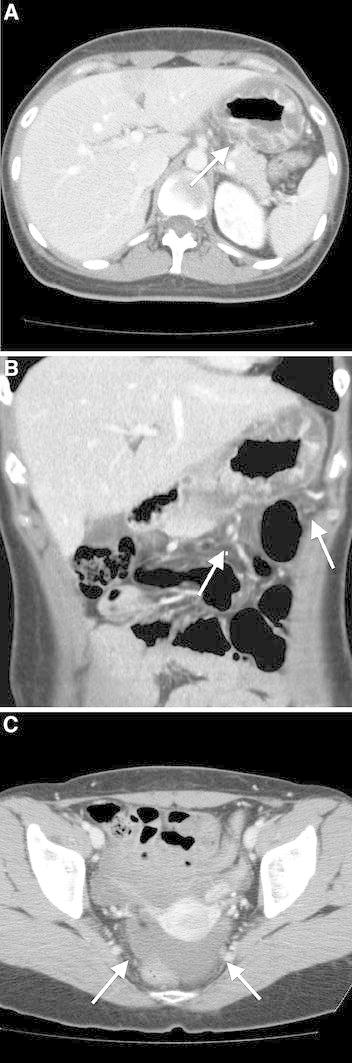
Gastric anisakiasis in a 32-year-old woman who presented with severe epigastric pain and a history of eating raw fish 1 day before symptom onset. A The CT image shows the severe mucosal edema of the whole gastric wall (*arrow*). B The coronal reconstructed image shows the edematous change of the stomach. Fat infiltration was also seen around the stomach (*arrow*). C A small amount of ascites was present in the pelvis (*arrow*).
